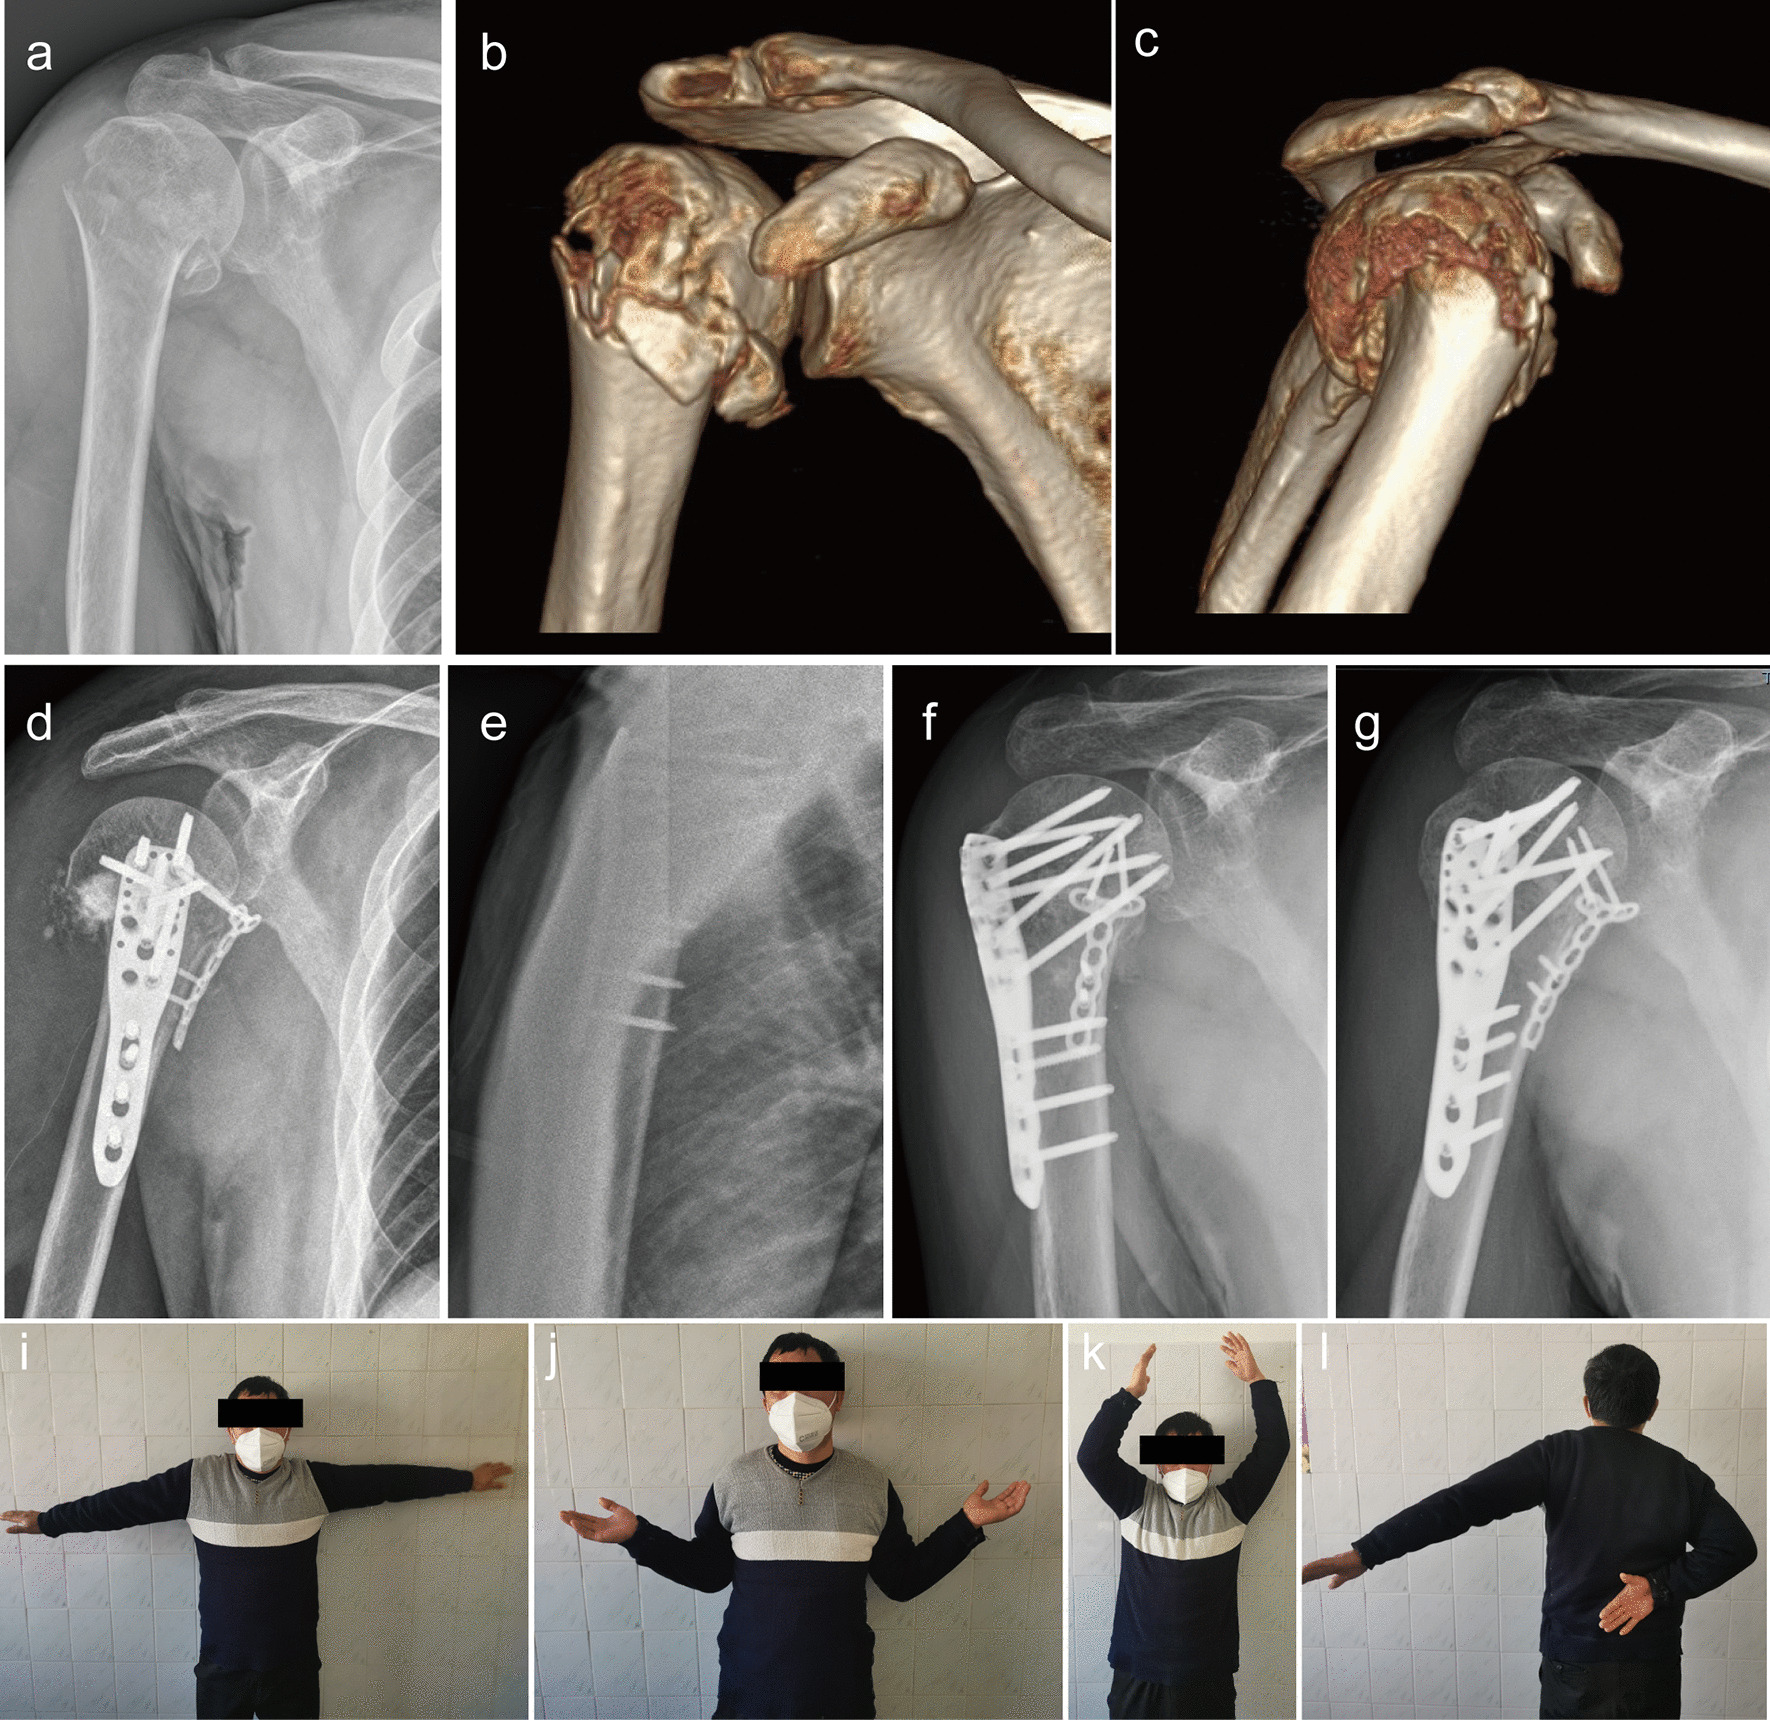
A 43-year-old patient with Neer 4-part proximal humeral fracture treated with the reduction technique. The AP (a) radiograph demonstrating displaced proximal humeral fracture, with the lateral radiograph unperformed due to severe pain. The CT reconstruction image (b, c) displaying a Neer 4-part fracture without any medial support. This patient was treated with PHILOS combined with a small anteromedial locking plate (d, e). X-ray images (f, g) at 11-weeks post-operation showing a bony union of the fracture. Clinical function was satisfactory at 1 year after the surgery (i–l)
